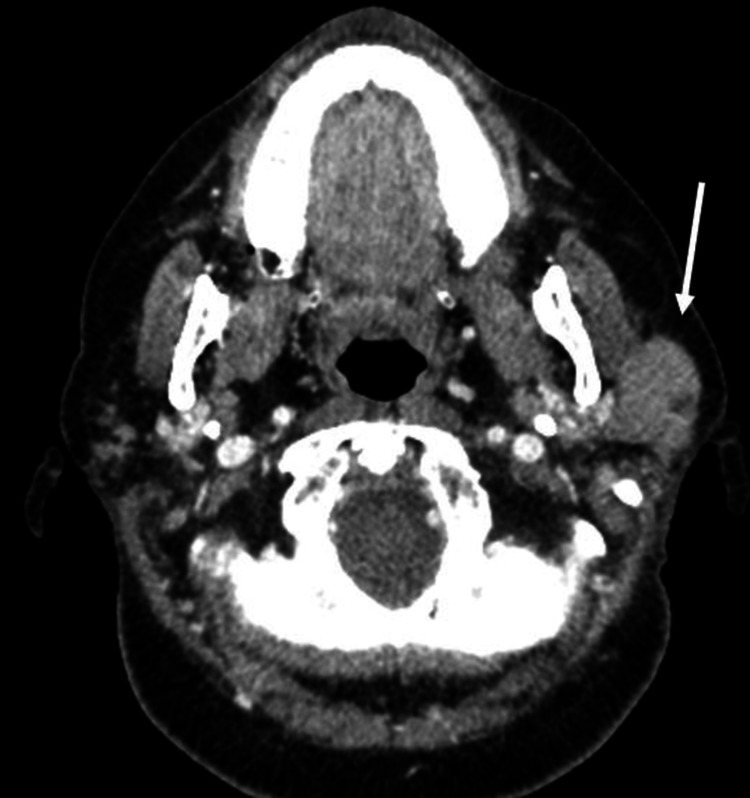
Axial CT image of the neck with intravenous contrast at the level of the parotid glands showing asymmetric left parotid gland enlargement with replacement by a soft tissue mass (white arrow).
